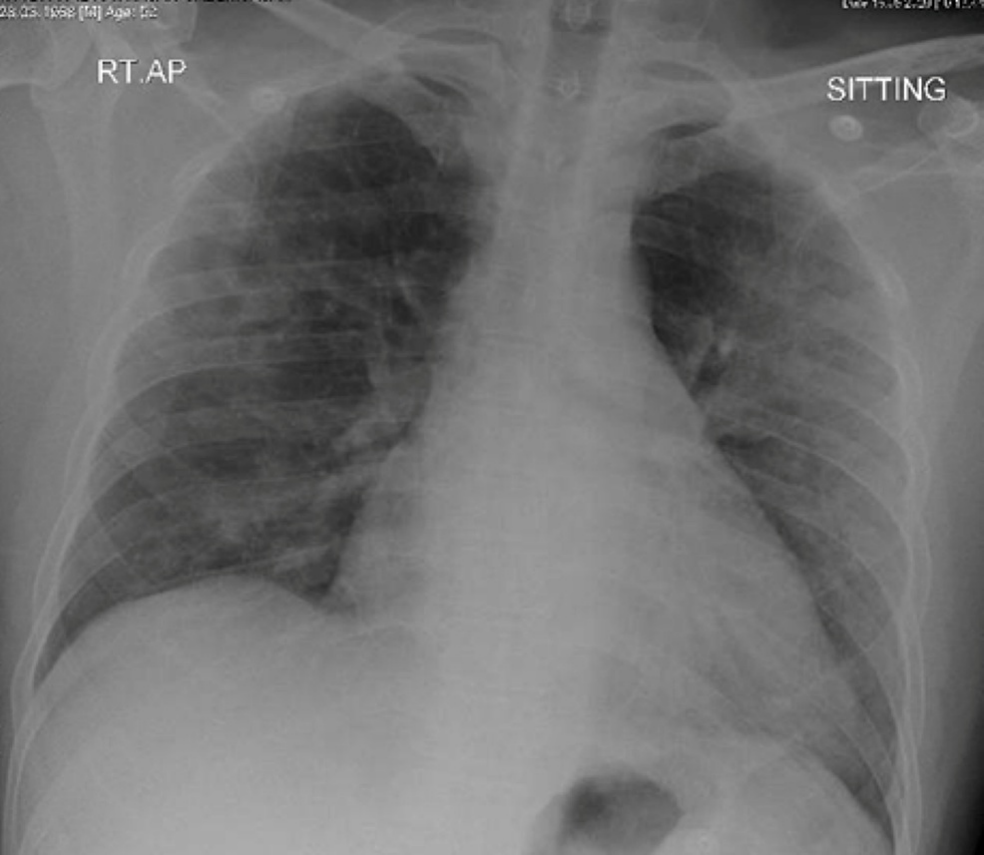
Bilateral predominantly peripheral alveolar infiltrates with loss of left hemidiaphragm silhouette on anteroposterior chest X-ray
